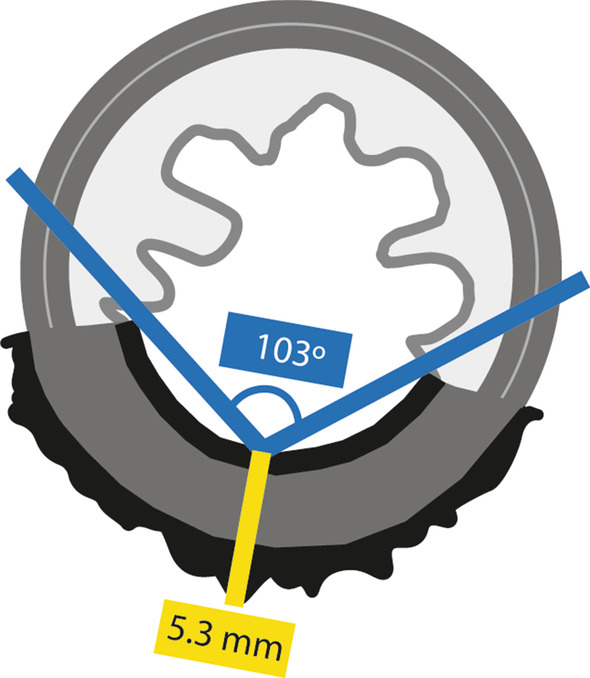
Scar depth angle and thickness measurements should be performed on the axial central slice of the tumour. For scar depth angle measurements of small (< 180°) tumour scars, the endoluminal centre of the scar serves as pivot while the angle lines cross the endoluminal extremities of the scar (in blue). In > 180° scars, the pivot should also be central but the angle lines should cross the endoluminal aspect of the scar at 180° of the rectal circumference and no more than that. The same applies to circumferential scars but in such cases, the pivot should be placed at the most invasive tumour front if there is one, or randomly if there isn´t. The most important thing to keep in mind is measuring at the exact same location in both first and second post-NAT MR imaging examinations. Scar thickness should be measured at pivot level, at the second MR imaging assessment. Measurement corresponds to a line that transverses tumour scar in depth, perpendicularly to it (in yellow), from inner to outer surface, irrespectively of it signal intensity
